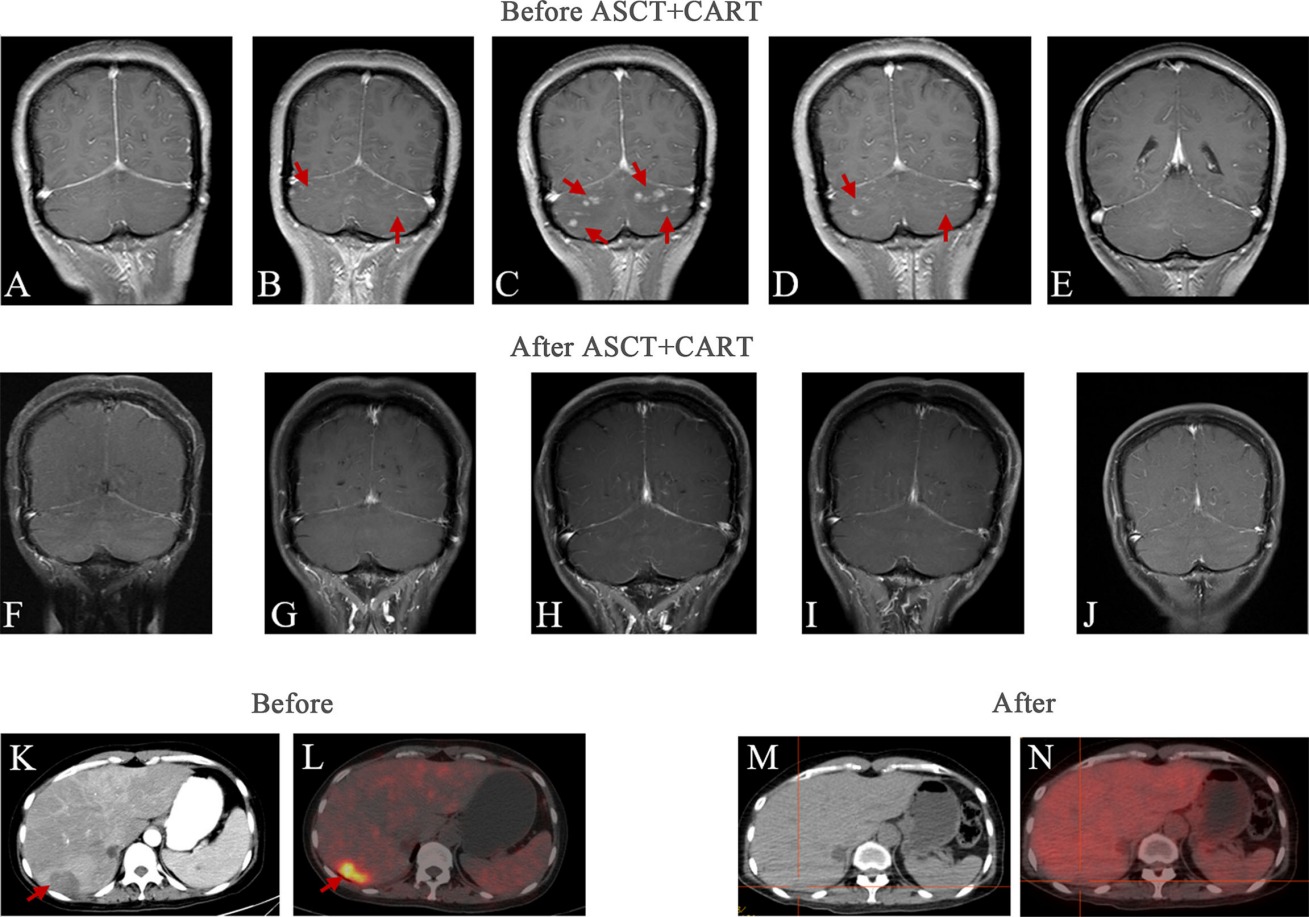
Head and liver imaging results of relapsed/refractory intravascular large B-cell lymphoma. Brain MRI images: (A) First time headache symptoms appeared at the initial diagnosis. (B)The intracranial infiltration was diagnosed. (C) 1 month after intrathecal chemotherapy. (D) 1 month after two courses of the adjusted treatment regimen MTX combined with Ara-C therapy. (E) 1 month before ASCT and CAR T-cell therapy. (F) 3 months after ASCT and CAR T-cell therapy. (G) 6 months after ASCT and CAR T-cell therapy. (H) 9 months after ASCT and CAR T-cell therapy. (I) 12 months after ASCT and CAR T-cell therapy. (J) 22 months after ASCT and CAR T-cell therapy. Abdominal images: (K) Abdominal CT at initial diagnosis. (L) PET-CT at initial diagnosis. (M) The most recent Abdominal CT after therapy. (N) The most recent PET/CT after therapy.
